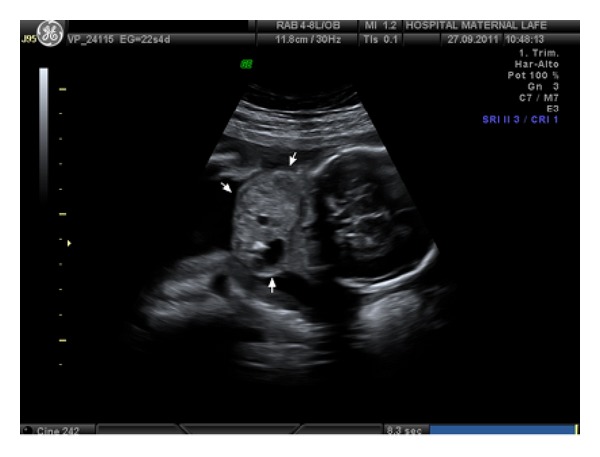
Ultrasound at 22 weeks, where heterogeneous tumor is found in cervical position, moderately vascularized.
